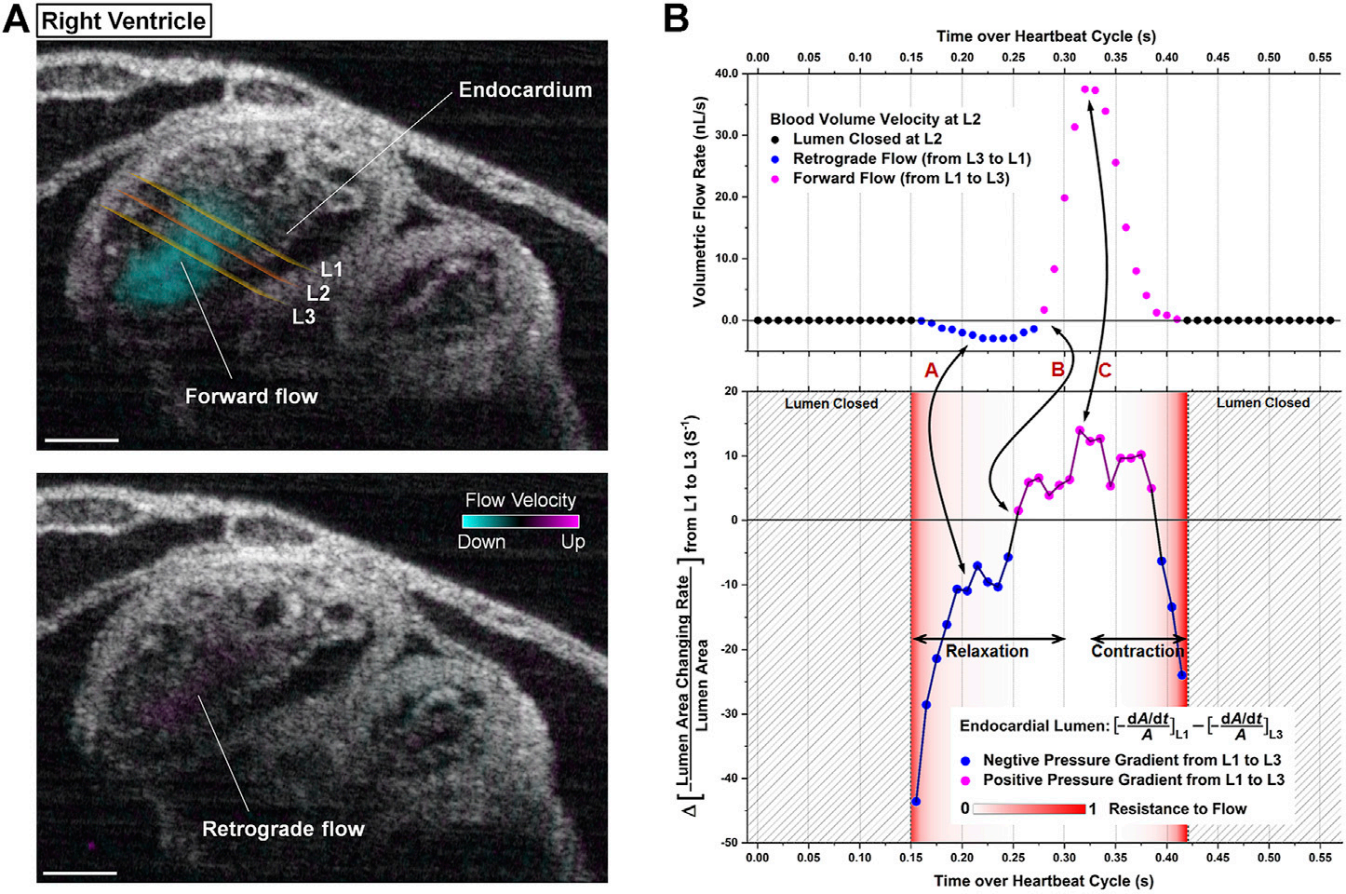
4D-OCT analysis of cardiac pumping in the right ventricle of E9.25 embryonic heart. (A) Structural and color-coded Doppler 4D-OCT flow images of the beating heart. The positions of the measurement planes where the analysis was performed are labeled as L1, L2, and L3. (Scale bar: 100 µm). (B) Corresponding quantitative analysis defining the relation between pressure gradient, resistance to flow, and volumetric flow rate toward the localized pumping investigation. Reproduced from (Wang and Larina, 2020).
